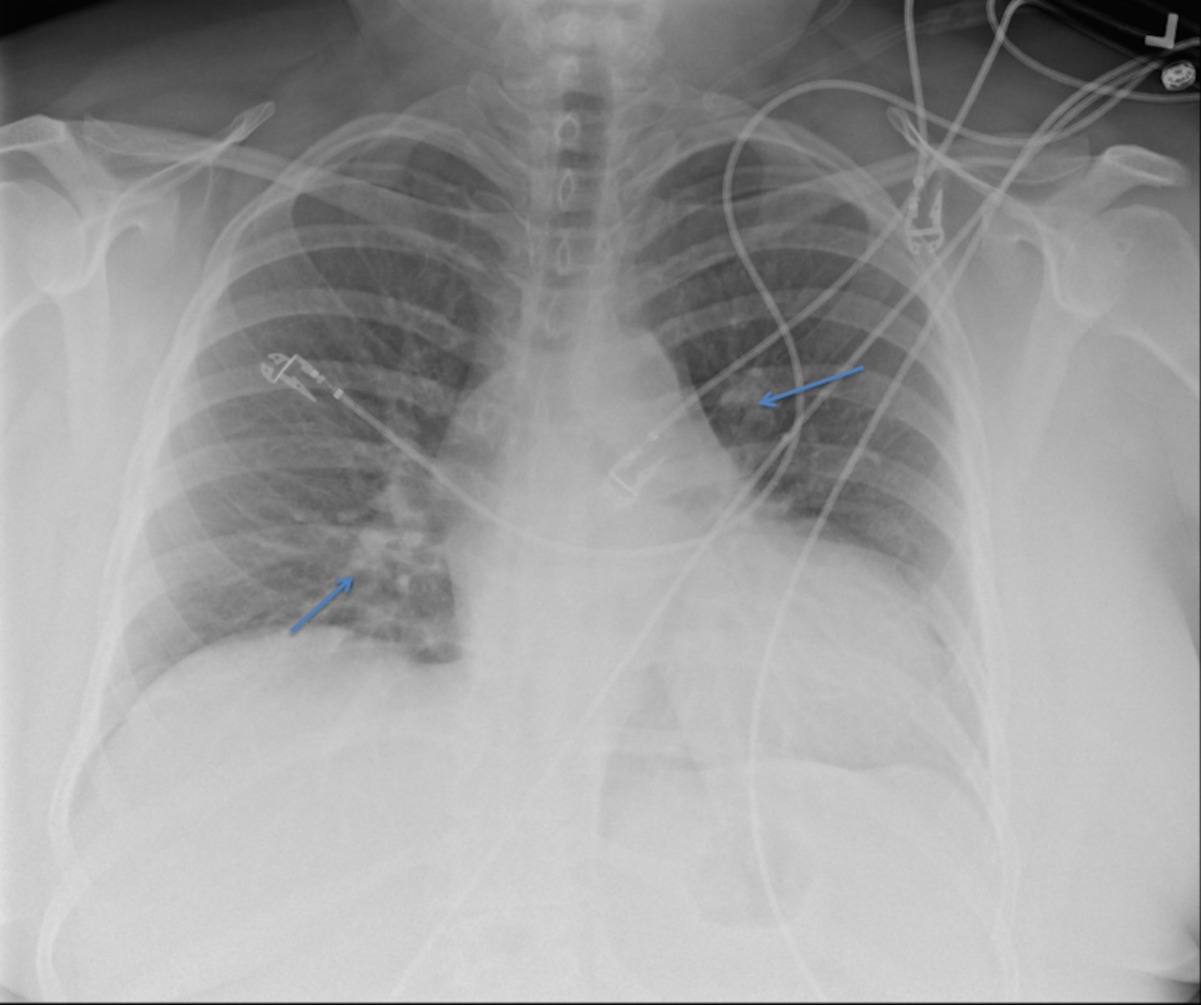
Chest X-ray Chest X-ray to identify pulmonary abnormalities. Note the asymmetric left suprahilar density and right infrahilar density in this image (arrows).
